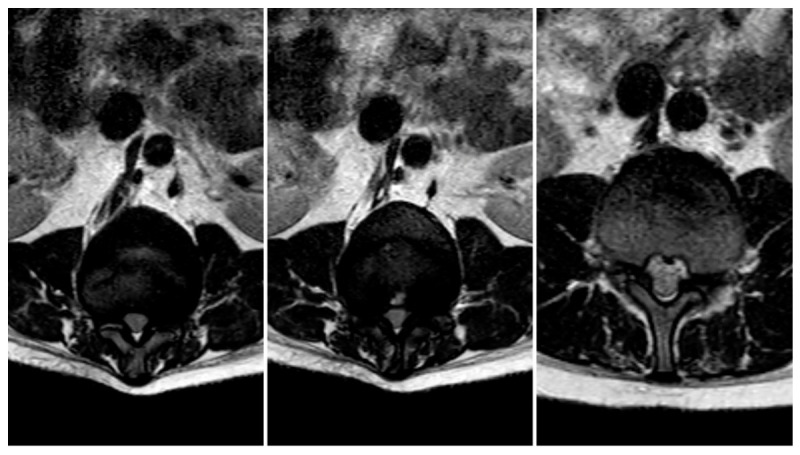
MRI of a 16-year-old girl with achondroplasia and congenital severe kyphosis before surgical treatment.
